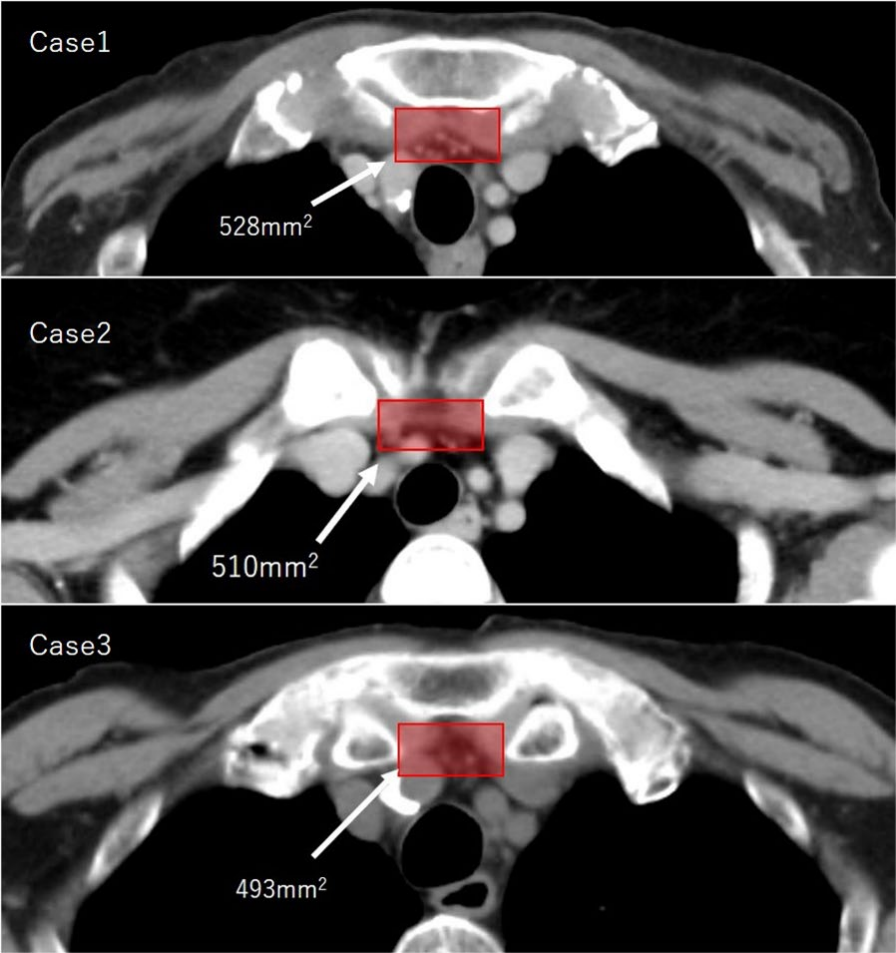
Measurement of the thoracic inlet space (TIS) in each case. The TIS was defined as the inter-clavicular distance multiplied by the sterno-brachiocephalic distance in the axial section of the cervical chest on preoperative computed tomography scans. In all the cases, the TIS was < 700 mm^2^
